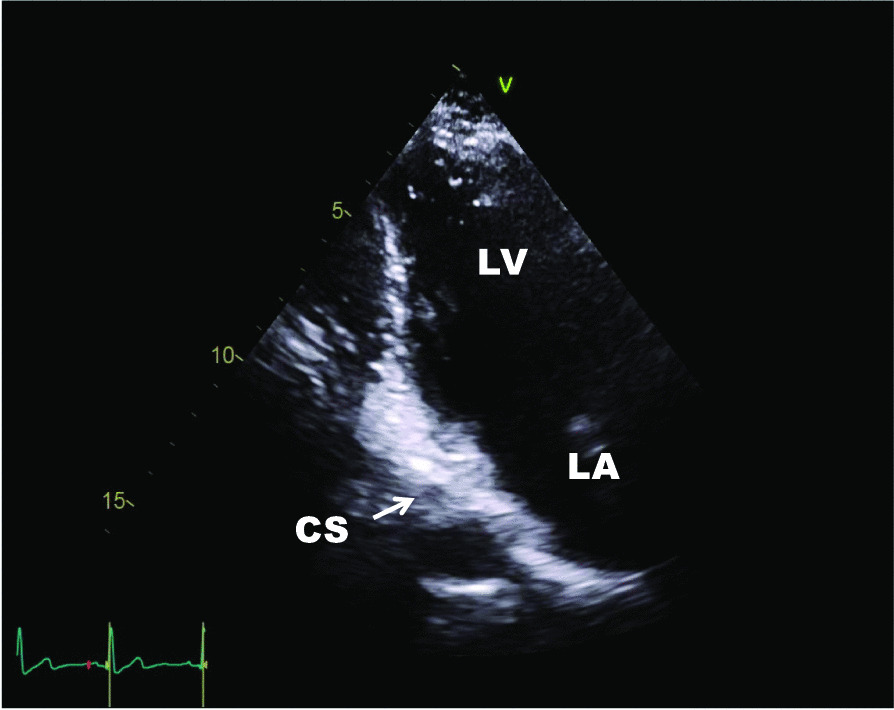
Transthoracic two-chamber view displaying the left side cavities (LA left atrium, LV left ventricle) without evidence of a dilated coronary sinus (CS, white arrow)
